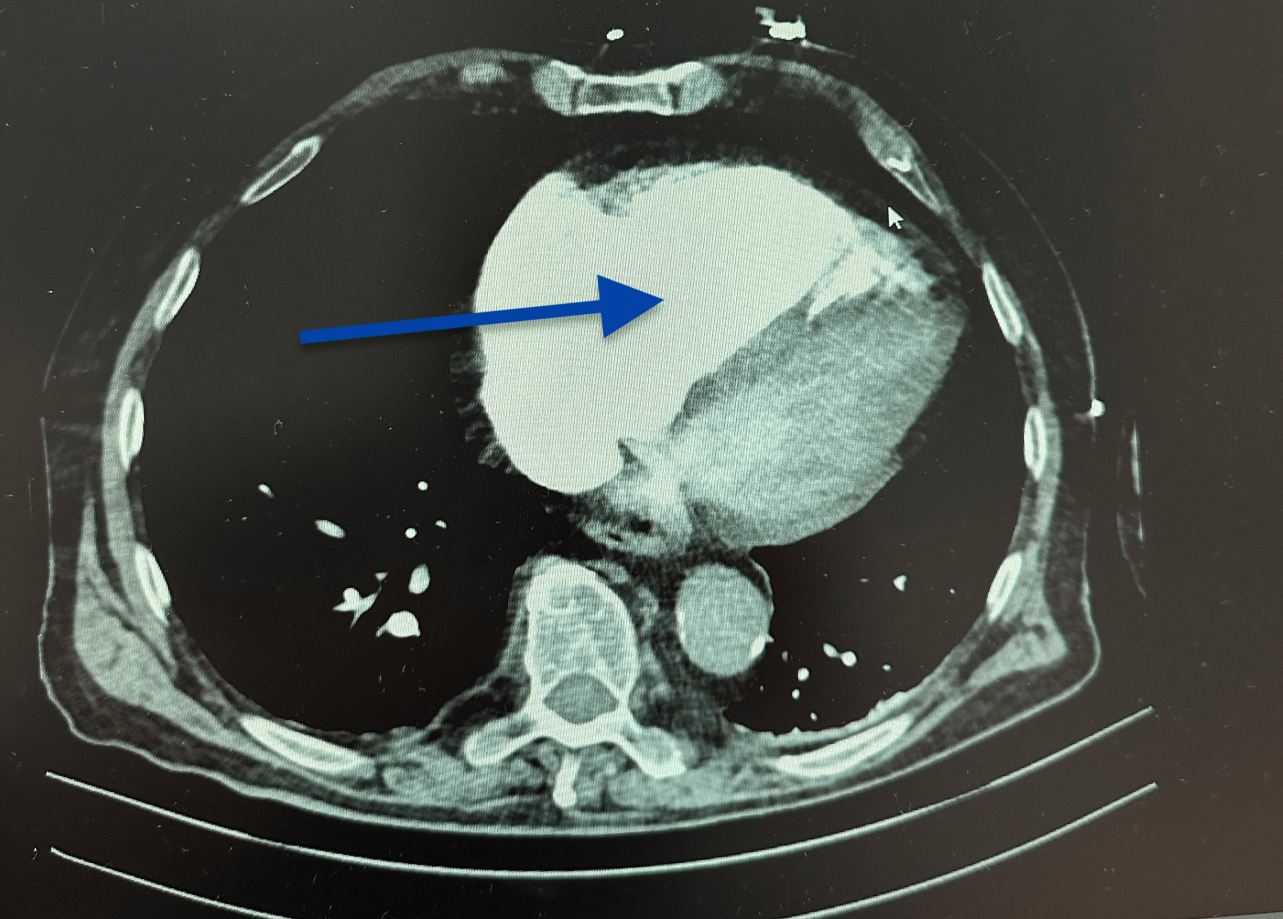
Right ventricular enlargement on computed tomography pulmonary angiography (CTPA). Right ventricular enlargement on computed tomography pulmonary angiography.
